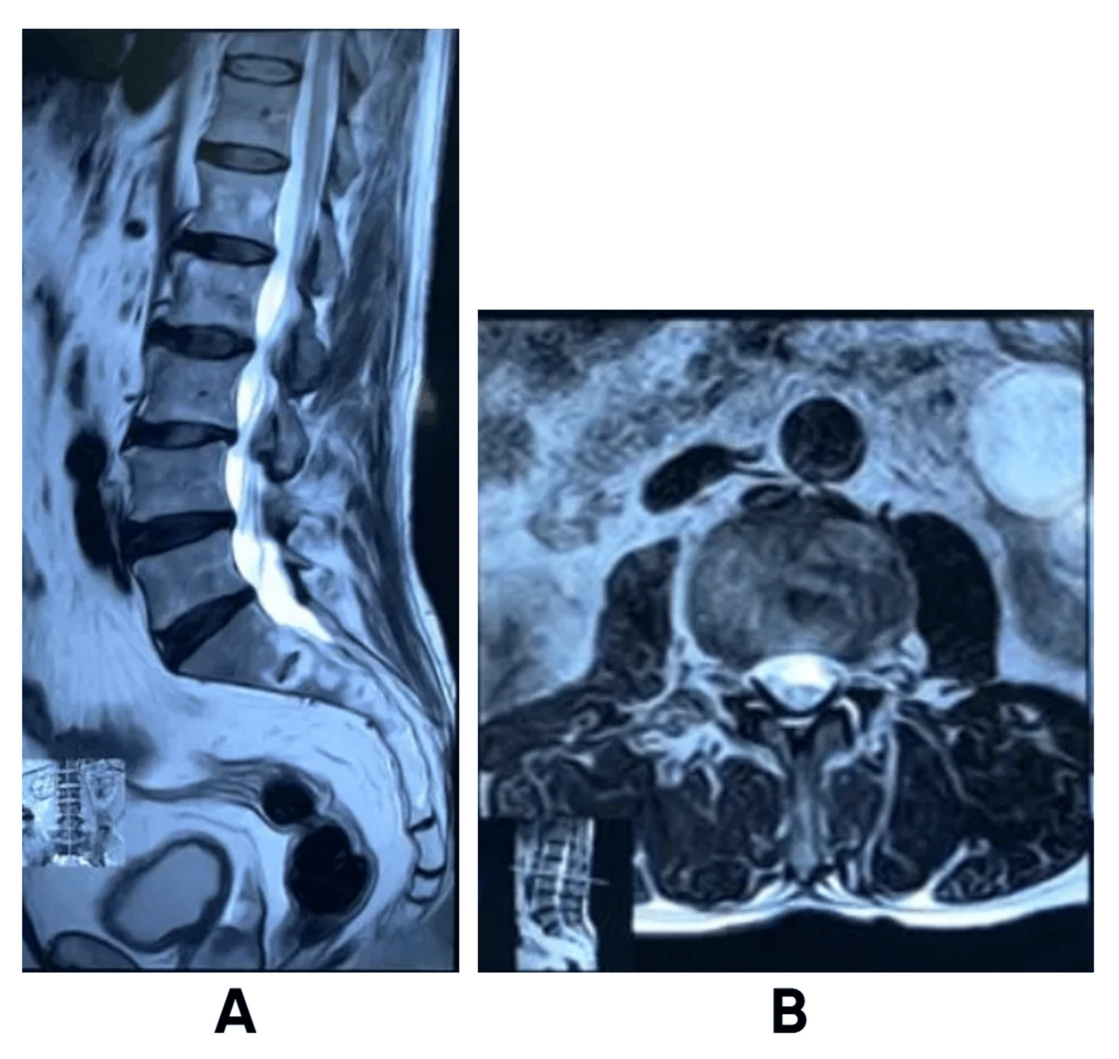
Preoperative lumbosacral spine MRI findings (A) Sagittal T2-weighted image showing preserved vertebral alignment with mild disc protrusions and no evidence of compressive or intradural pathology. (B) Axial T2-weighted image at the L4–L5 level demonstrating intact neural structures and absence of nerve root compression.
